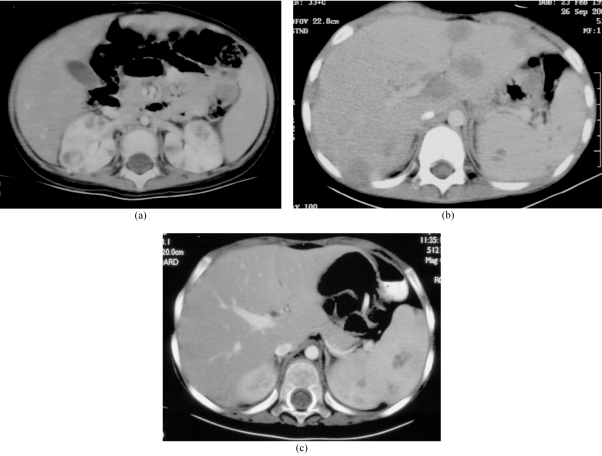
Fungal abscesses in intrabdominal organs. (a) A two-year-old girl with leukaemia and Aspergillus sepsis. CT showed multiple hypodense lesions in both kidneys. (b) A six-year-old girl with leukemia and presumed fungal sepsis. CT revealed multiple hypodense lesions in the liver and spleen. Fungal elements were isolated from liver biopsy, but the species could not be identified. (c) A three-year-old boy with leukemia with presumed fungal sepsis. CT abdomen showed multiple hypodense splenic lesions No fungi were isolated from biopsy.
